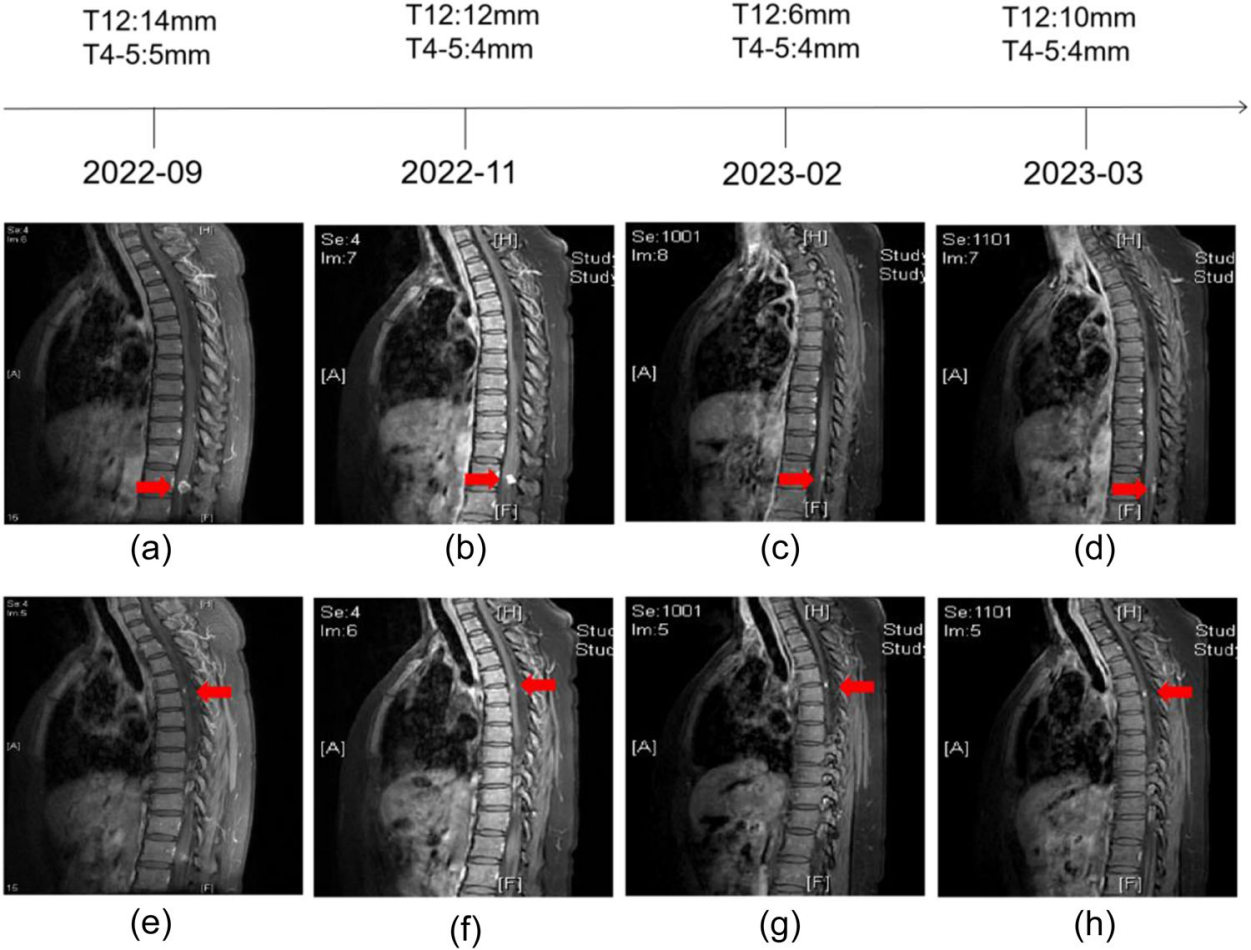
Spinal MRI of the patient during the whole treatment. The changes in the sizes of T4‐5 and T12 spinal HB during the whole treatment (a–h) can be observed. MRI, magnetic resonance imaging.
